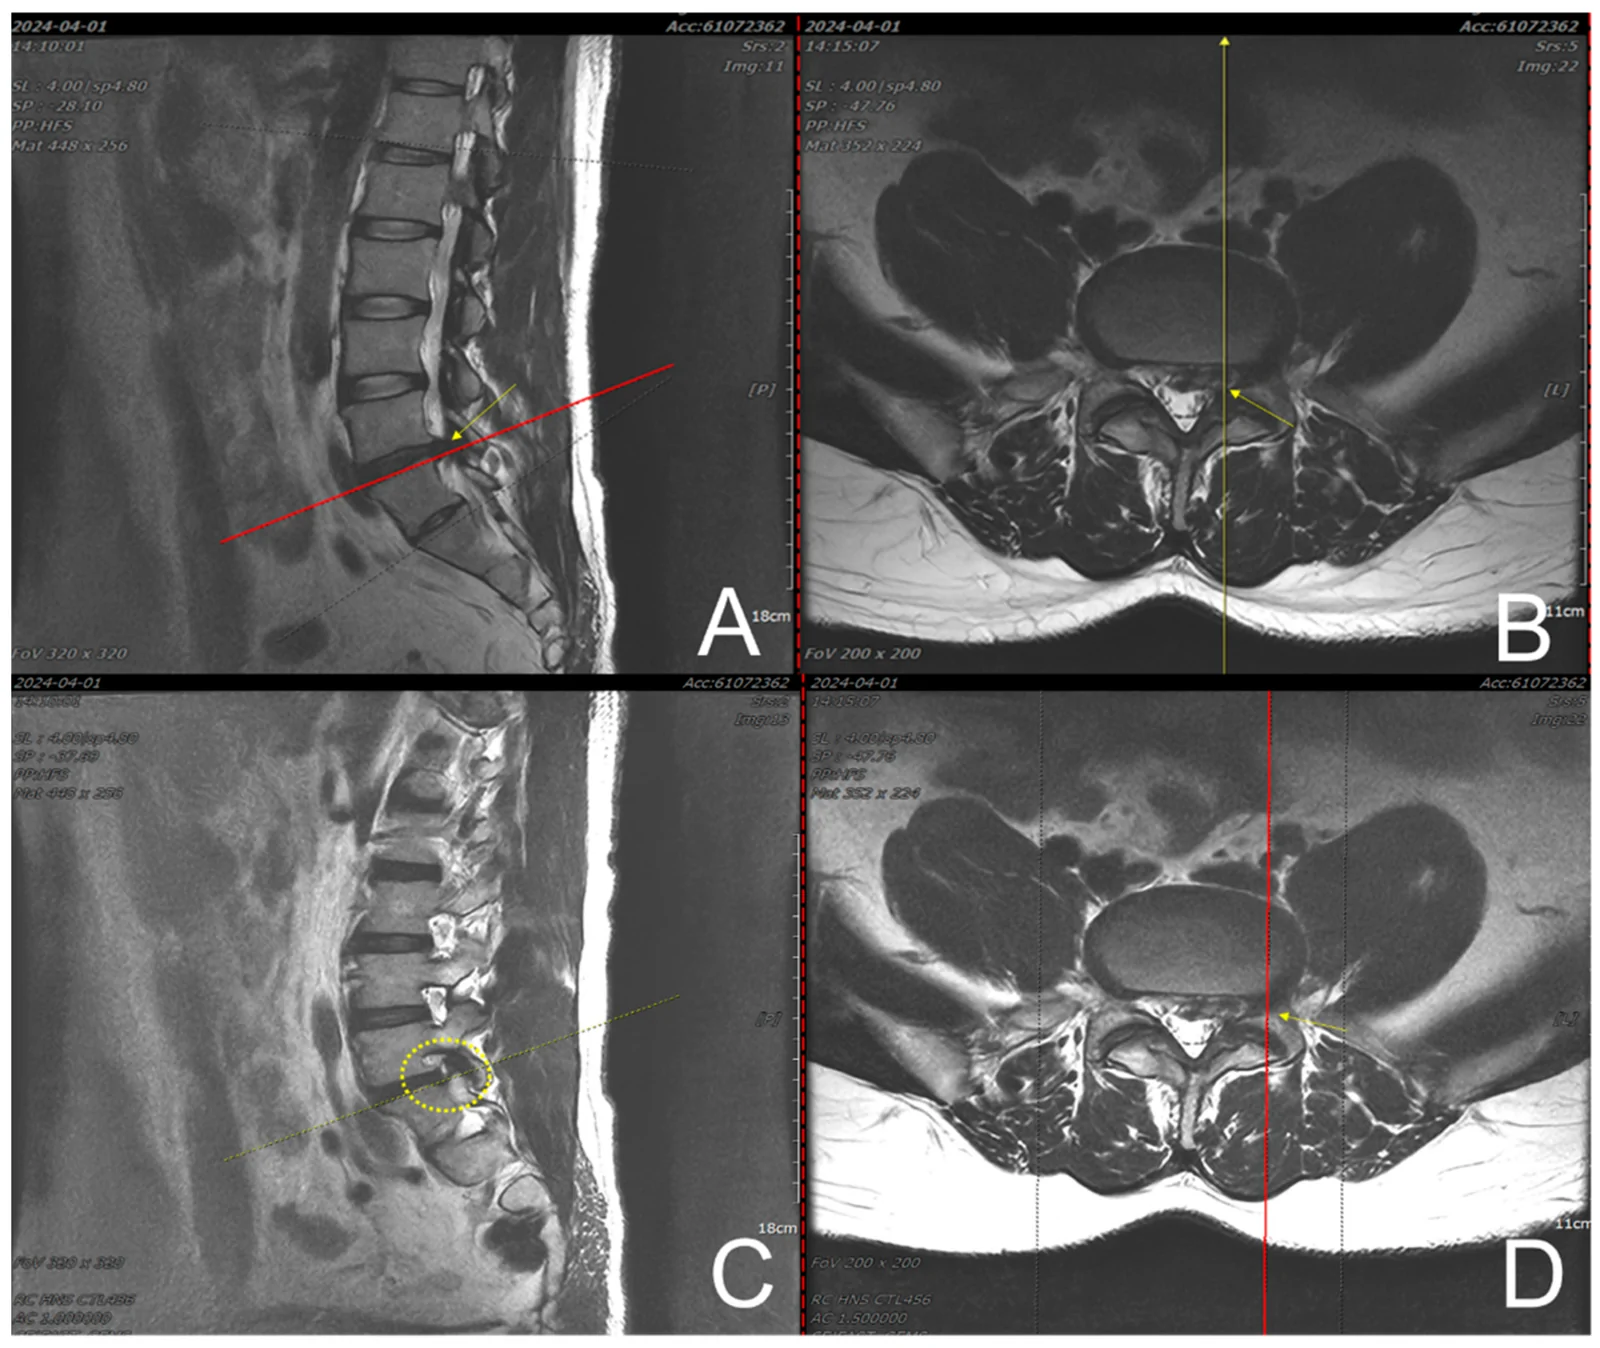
Lumbar spine MRI conducted on 1 April 2024. (A) T2-weighted turbo-spin-echo sagittal view. The yellow arrow indicates an extruded disc at the L5/S1 level. (B) T2-weighted turbo-spin-echo axial view. The yellow arrow indicates the compression of the left S1 traversing nerve root by a disc extrusion in the left paracentral zone at the L5/S1 level. (C) T2-weighted turbo-spin-echo sagittal view at the L5/S1 left extraforaminal zone. The yellow circle indicates a significantly compressed spinal canal compared with the upper levels. (D) T2-weighted turbo-spin-echo axial view at the L5/S1 left extraforaminal zone. The yellow arrow indicates the compression of the left L5 exiting nerve root by a disc extrusion in the left extraforaminal zone at the L5/S1 level. MRI, magnetic resonance imaging.
